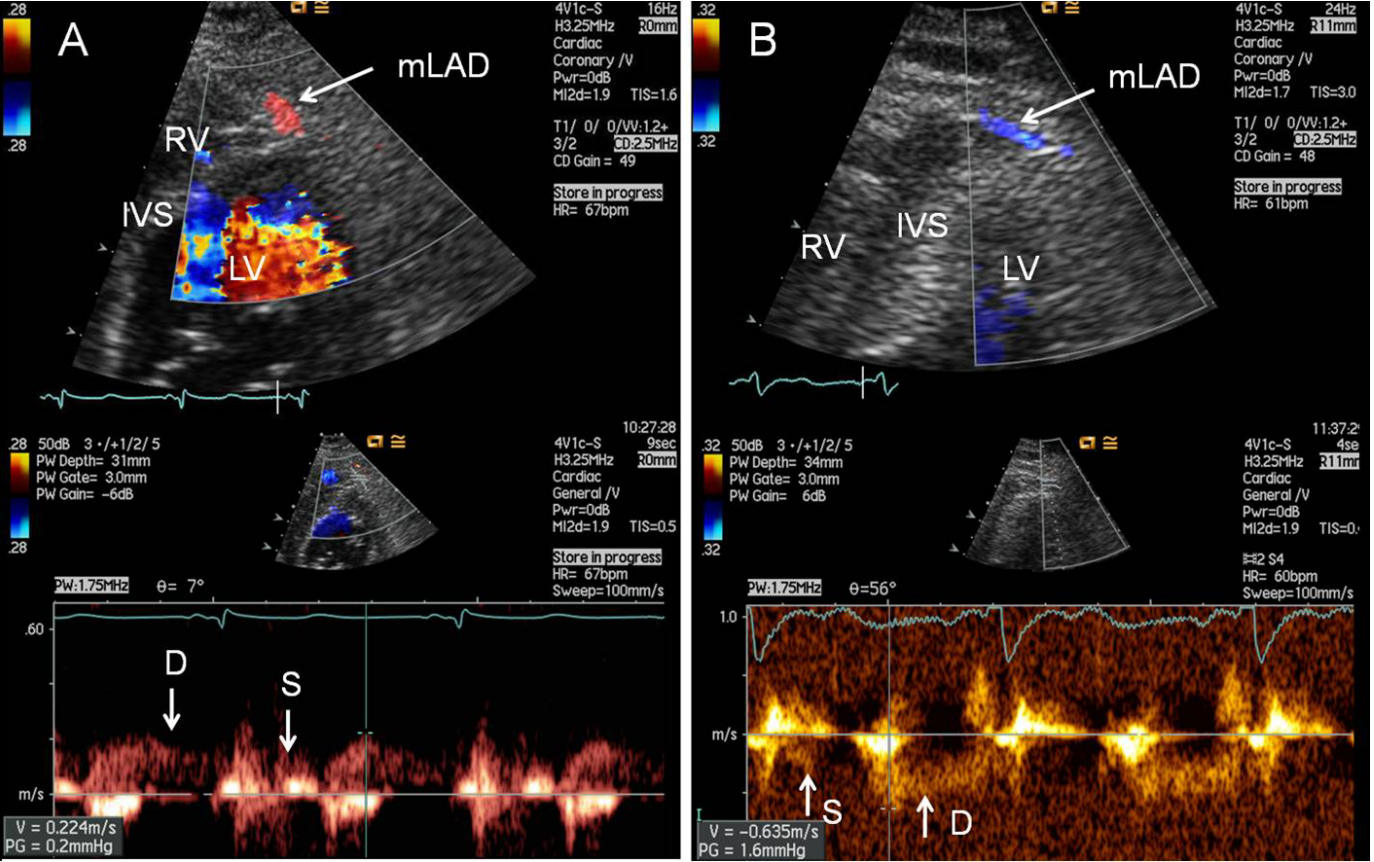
Examples of antegrade and retrograde flow in the middle segment of LAD. The middle segment of the left anterior descending coronary artery (mLAD) imaged by colour Doppler mapping and matching spectral Doppler tracings of blood flow, imaged from modified parasternal short-axis view focusing on the anterior interventricular sulcus: (A) The mLAD is seen with antegrade flow. (B) The mLAD is seen with retrograde flow. D = spectral Doppler tracings of diastolic coronary blood flow; IVS = interventricular septum; LV = left ventricle; RV = right ventricle; S = spectral Doppler tracings of systolic coronary blood flow.
